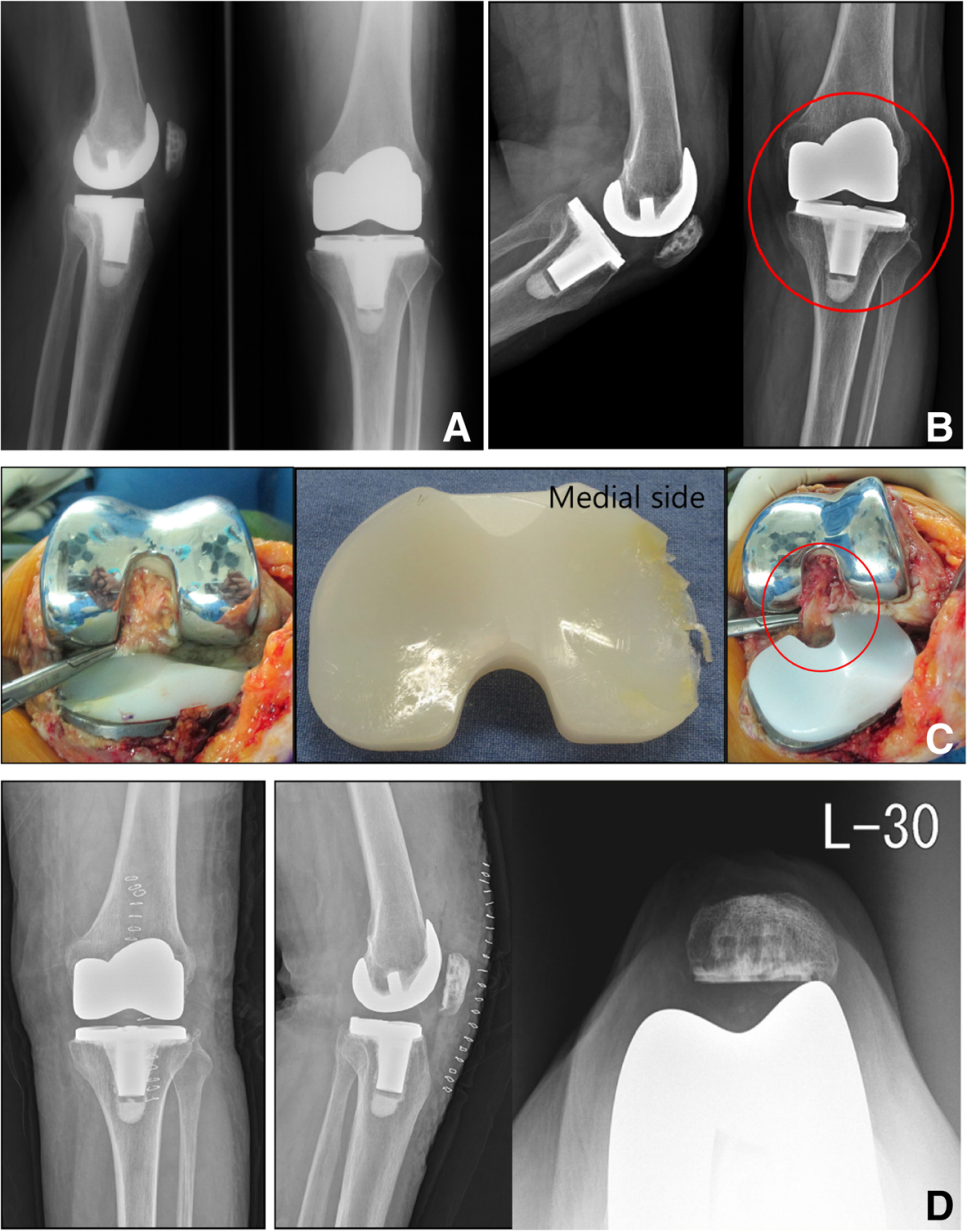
a A 63-year-old woman with osteoarthritis. Postoperative radiograph. b Radiograph taken 15 years after surgery shows wear of meniscus bearing. c Intraoperative finding shows wear of meniscus bearing and an intact posterior cruciate ligament, so isolated meniscus bearing was diagnosed. d Radiograph taken after revision surgery
